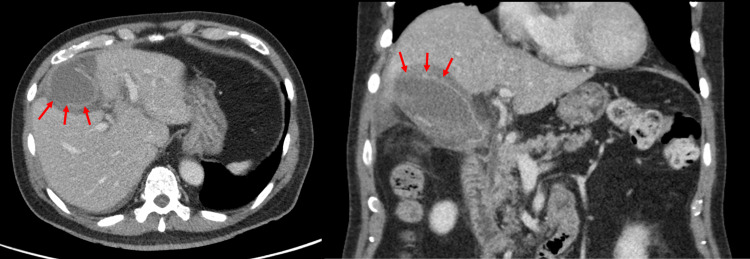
Transverse and coronal CT images of Case 2 showing gallbladder oedema, peri-cholecystic fat stranding and discontinuity of mural enhancement (arrows).
